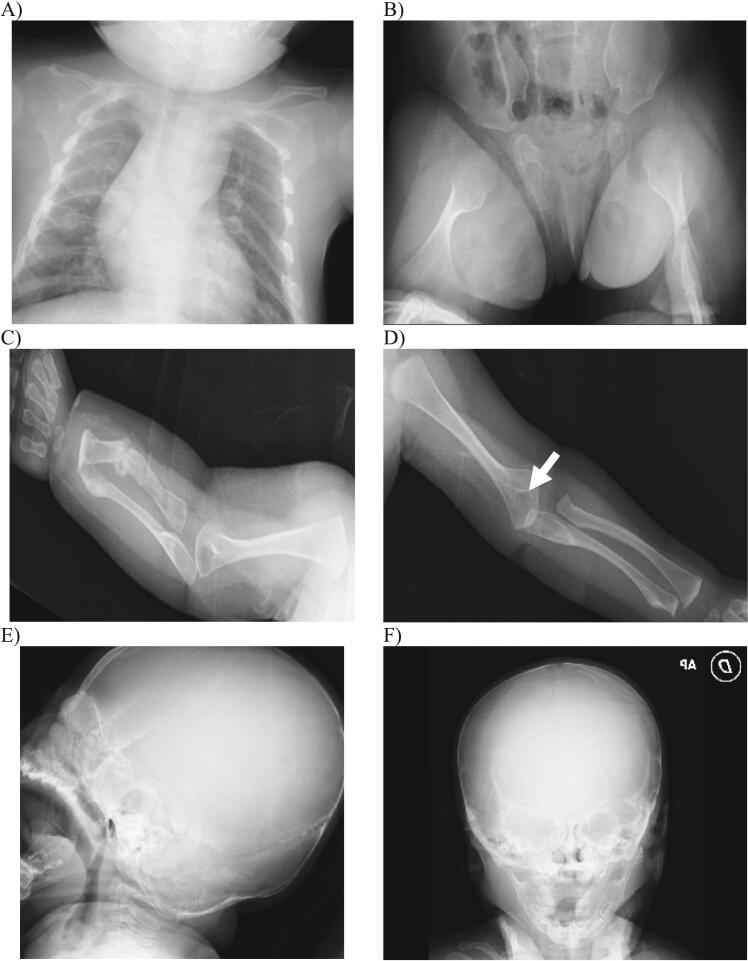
A) Chest X-ray showed callus formation in the 4th, 5th, and 6th left costal arches, as well as the 6th and 7th right costal arches and left clavicle, indicating probable fractures occurring during the prenatal stage. B) Pelvic X-ray revealed a distal metaphyseal fracture of the left femur, angled dorsally at 40°, without involvement of the joint. Pathological widening of the metaphysis was noted, along with overall osteopenia. C) X-ray of the upper right extremity displayed an old diaphyseal fracture of the forearm with bone callus formation. Additionally, a new fracture of the distal radial metaphysis was observed, with the patient wearing a splint at the time of evaluation. D) X-ray of the upper left extremity revealed an old, non-displaced, and non-angulated distal metaphyseal fracture of the humerus. E and F) Cranial X-rays revealed overall demineralization in the diploe, along with Wormian bones in the lambdoid suture.
